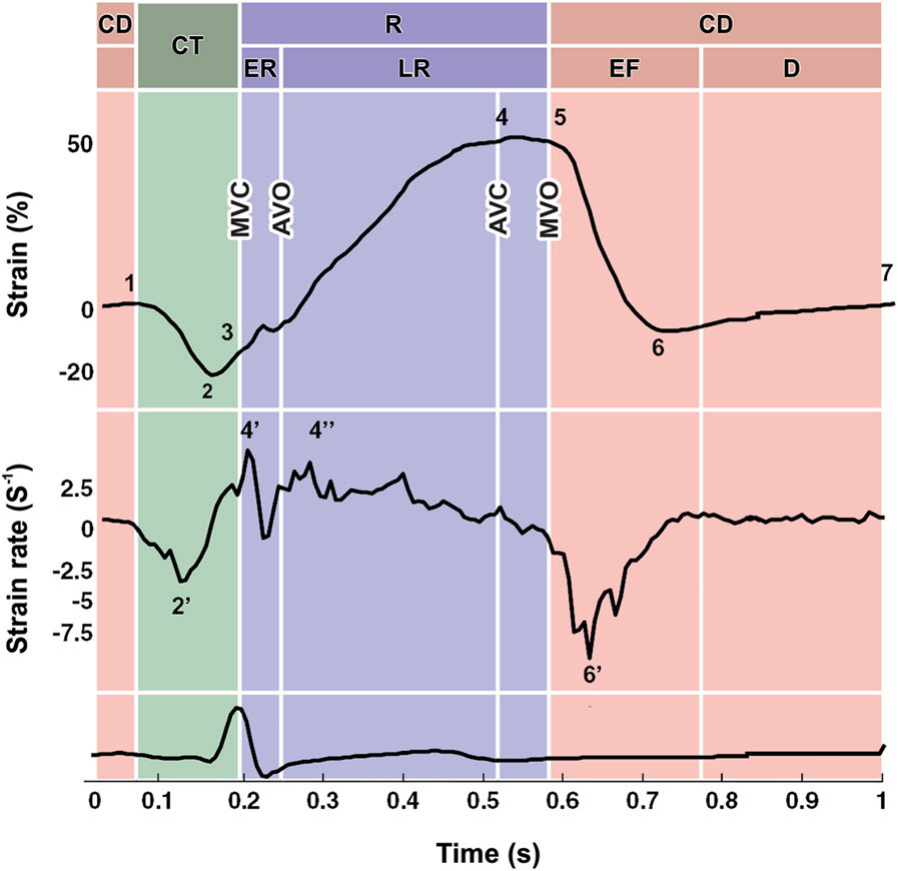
Normal strain and strain rate echocardiography curves in normal subjects. Atrial periods: CD = conduit period; CT = contraction period; R = reservoir period
